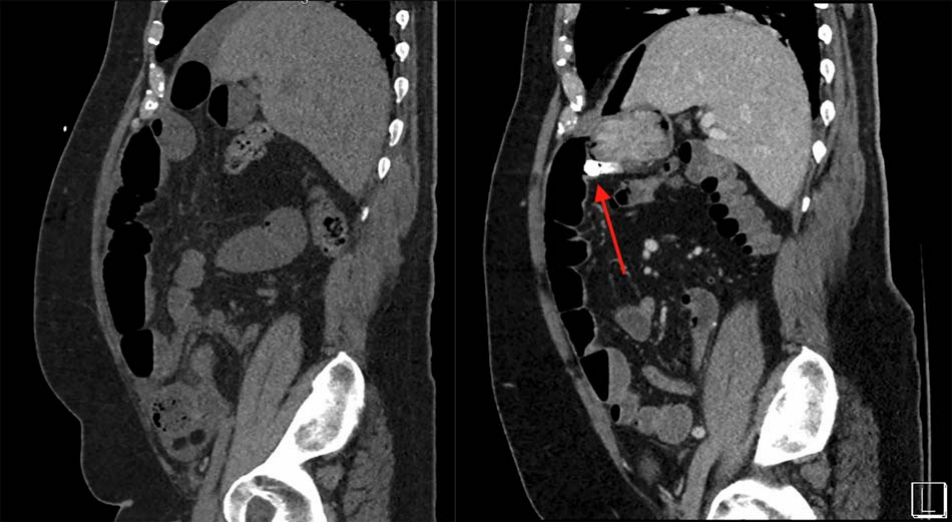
On the left is the initial CT scan non-contrast demonstrating no obvious mass. On the left is the CTA, a week later, showing a mass and pill cam in the right upper quadrant.
